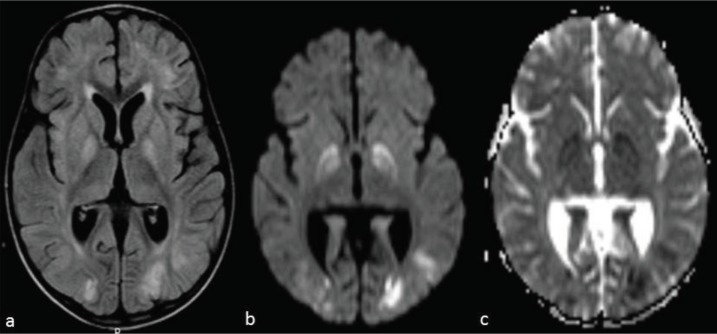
year old boy after liver transplantation (patient 1). Axial MR image a. (fluid attenuation inversion recovery) demonstrates involvement of bilateral globus pallidi and frontal and occipital lobes. Diffusion-weighted images b. with b-1000 and c. with apparent diffusion coefficient demonstrate decreased diffusion in the occipital lobes and globus pallidus
